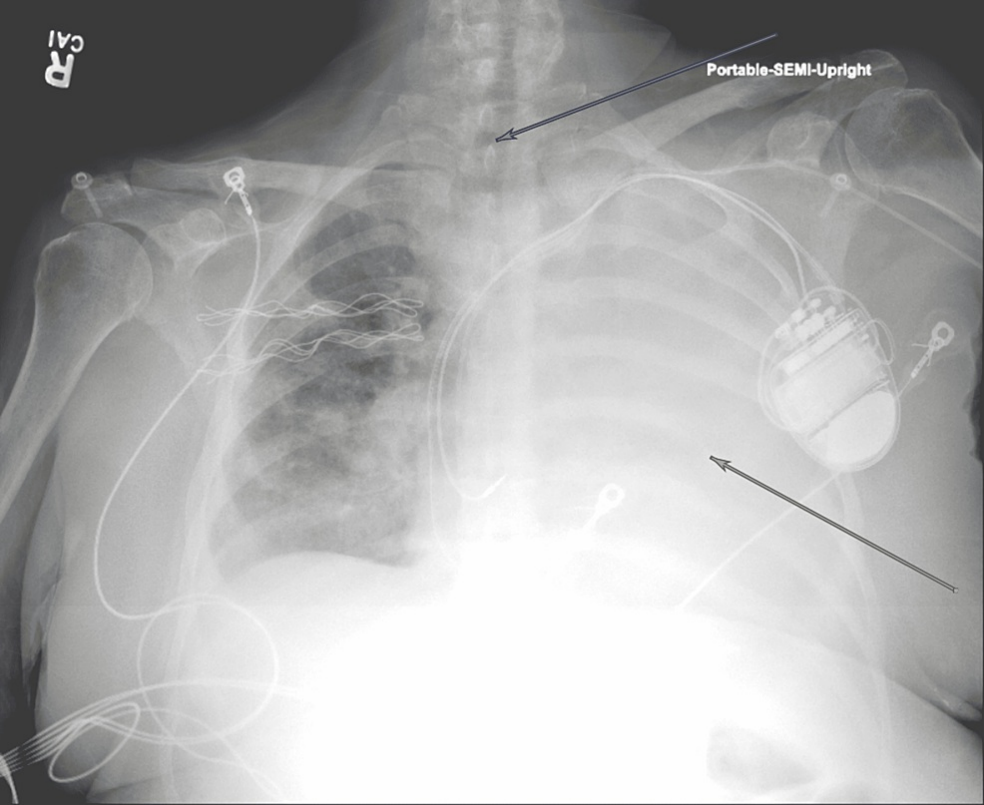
Initial chest X-ray upon hospital transfer demonstrating complete opacification of the left thorax (yellow arrow) with slight right-sided mediastinal shift (blue arrow)
